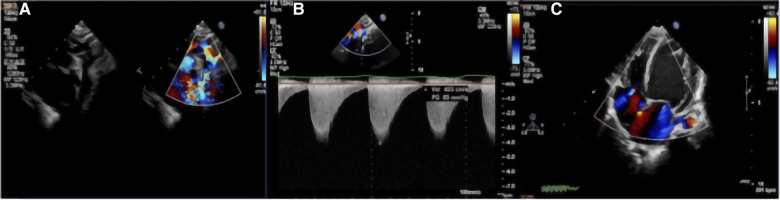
TTE with color Doppler flow imaging showed the location of the CoA and increased blood flow velocity: (A) indicate the location of the CoA and multicolored flow signal; and (B) increased blood flow velocity with a high-pressure gradient, which was estimated by a continuous-wave Doppler. (C) Left ventricular hypertrophy.
